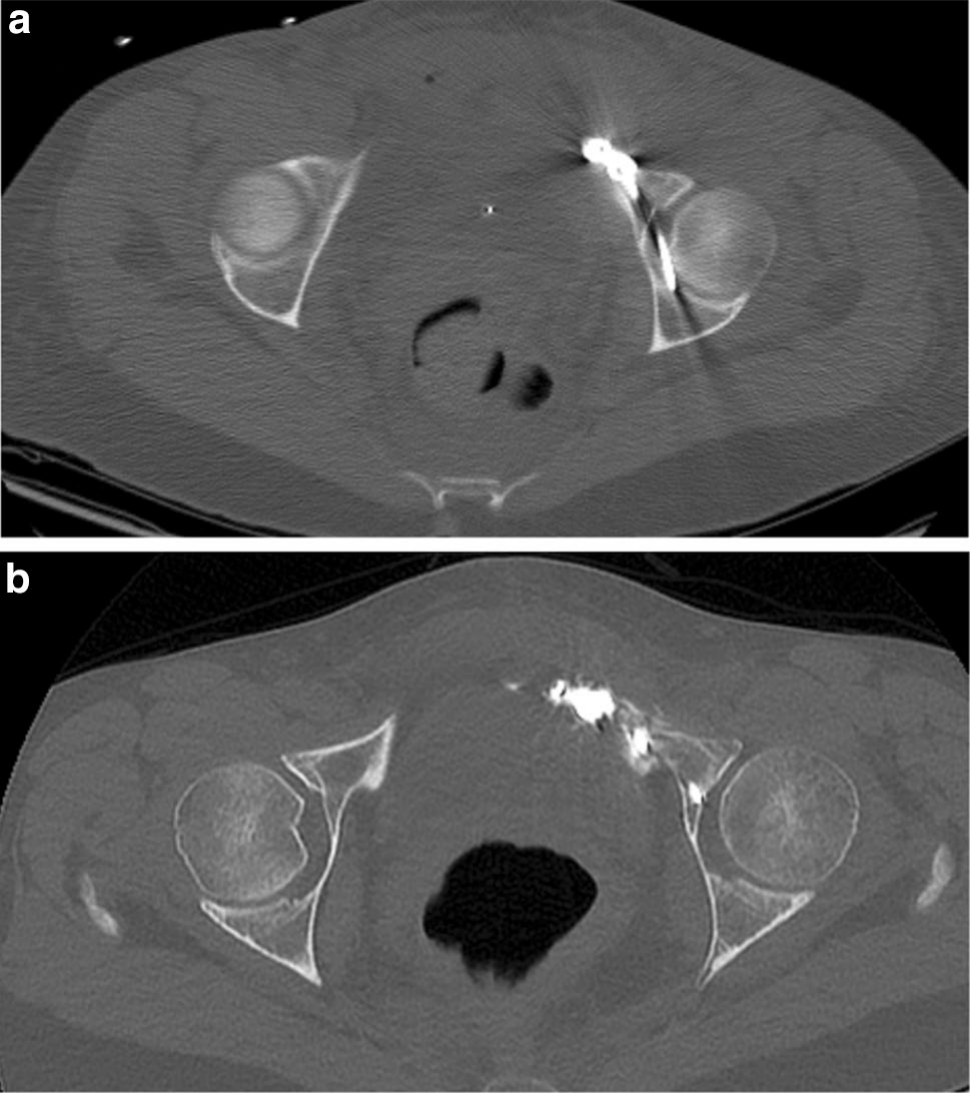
a Postoperative CT-scan in a 48-year female with a lateral compression type fracture displaying a screw penetrating the left acetabulum. b Postoperative CT-scan in the same patient after reoperation with exchange of the screw
